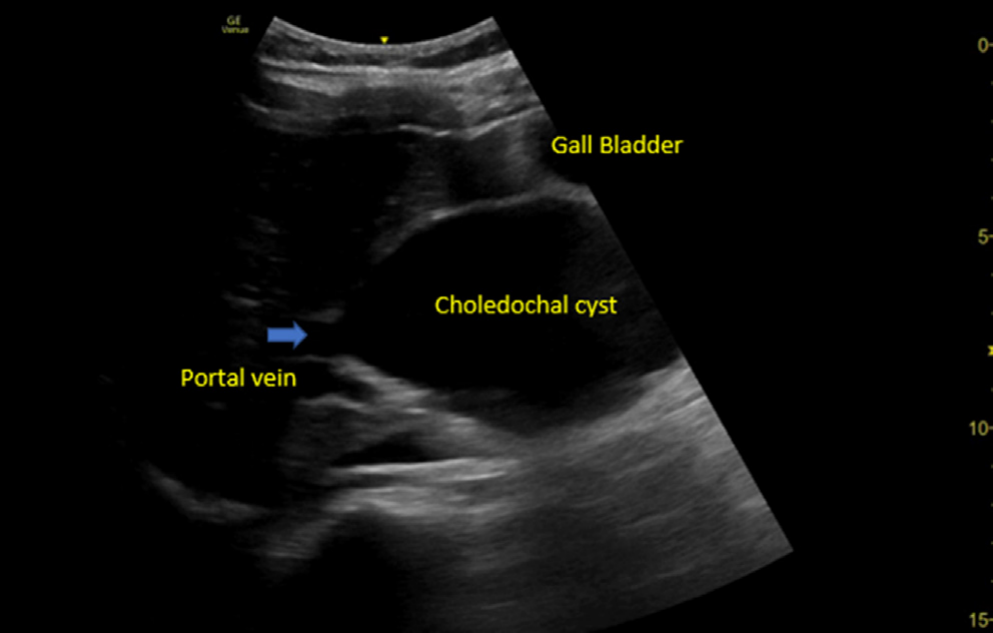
Point-of-care ultrasound showed an evaluation of the common bile duct (blue arrow), which lies above the portal vein and continues with the choledochal cyst.
